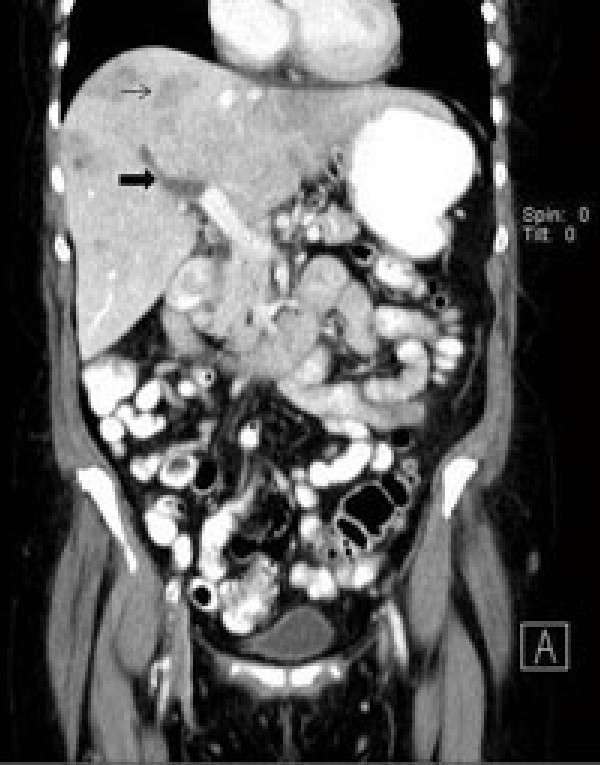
Computed tomography (CT) scan scout view showing right portal vein thrombus and liver abscess.
